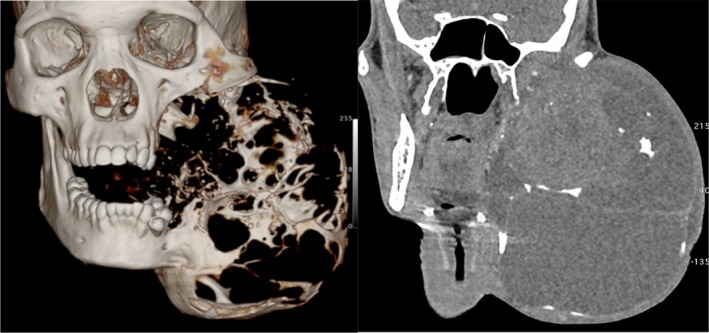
3‐D reconstructed CT scan and coronal view demonstrating the tumor extent
